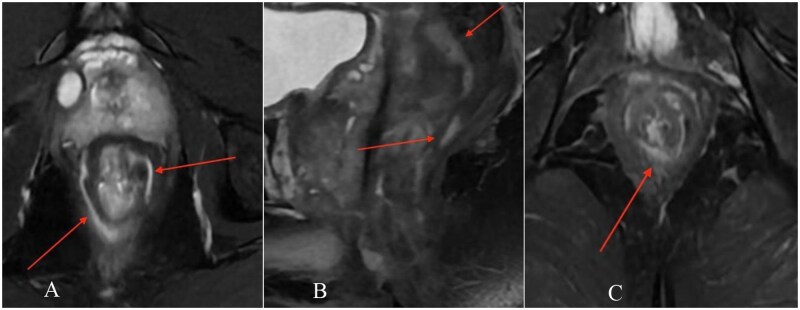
Circumferential perirectal inflammatory collection with extensive pelvic involvement. (A) Axial T2-weighted image demonstrates circumferential hyperintense perirectal collection surrounding the rectum, with associated mural thickening and inflammatory change (arrows). (B) Sagittal T2-weighted image shows longitudinal extent of the perirectal inflammatory process and associated mesorectal/perirectal edema (arrows). (C) Coronal fat-suppressed T2-weighted image depicts the circumferential perirectal collection and surrounding inflammatory change in the lower pelvis (arrow).
